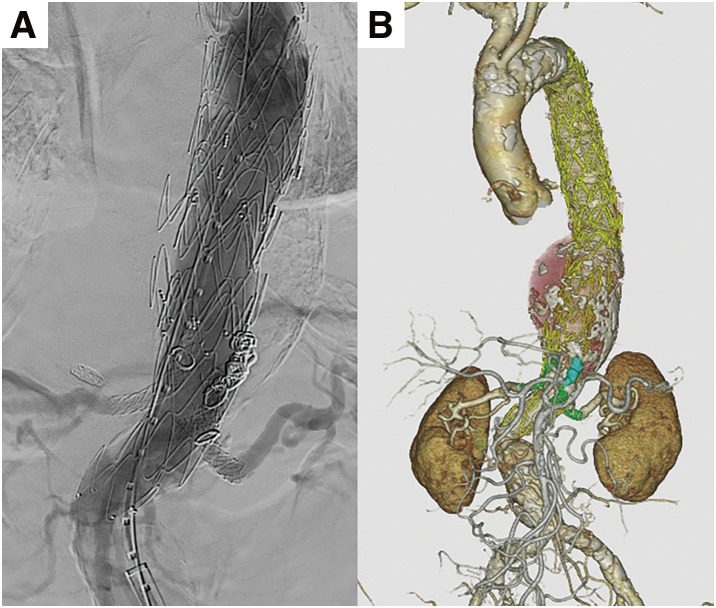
Angiography following device implantation. (A) Final digital subtraction angiography during the second surgery. (B) 3D-CT angiography after the second surgery.
